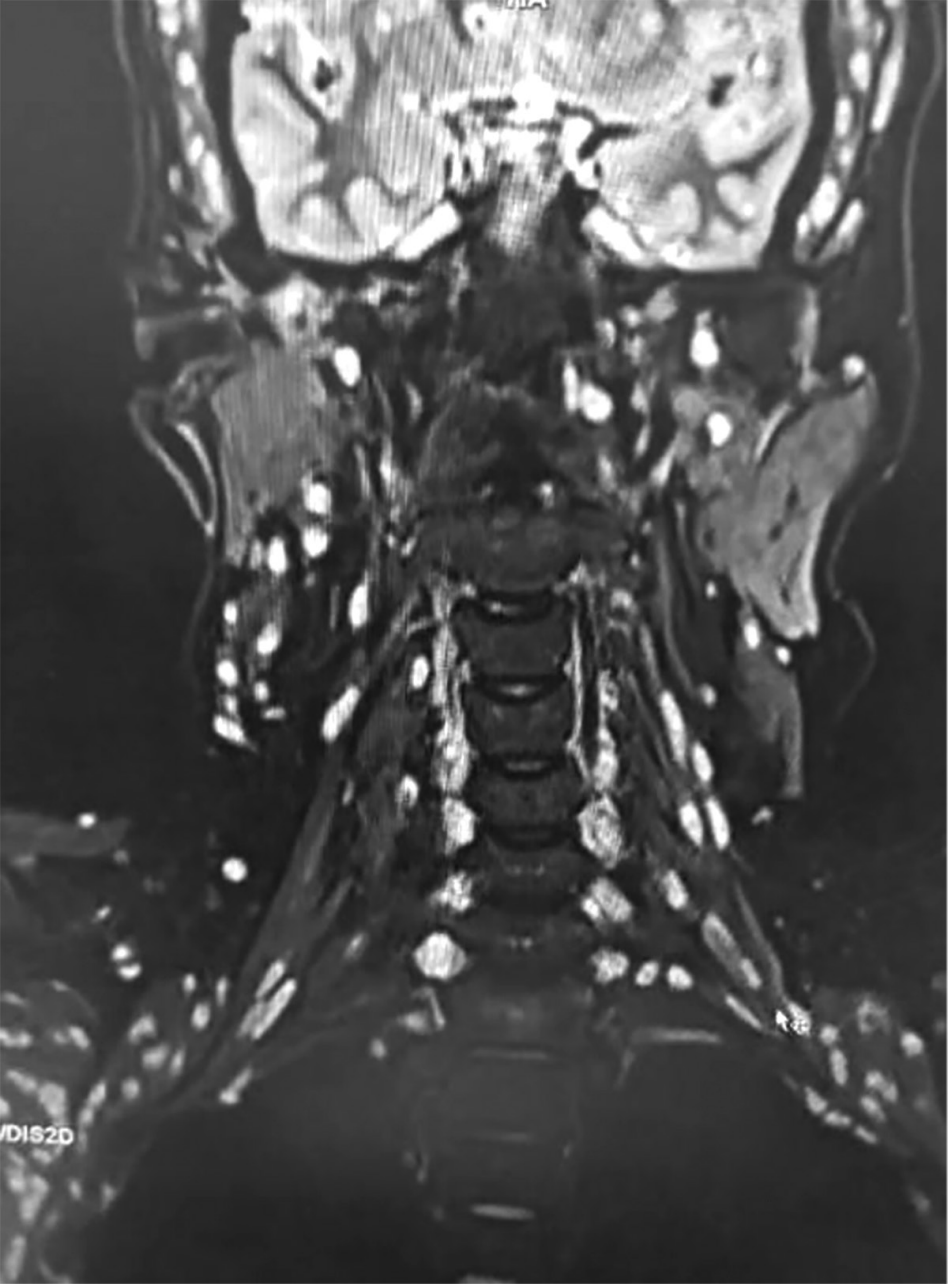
Description: Coronal T2-weighted MRI of the cervical spine and neck muscles shows multiple and well-defined cystic lesions distributed symmetrically within the paravertebral and posterior cervical musculature. Several lesions demonstrate an eccentric hyperintense focus representing the scolex, consistent with myocysticercosis. The cervical vertebral bodies and intervertebral discs are preserved, and no compressive myelopathy is seen. Findings are characteristic of disseminated muscular cysticercosis involving the cervical region. Clinical Significance: This imaging highlights the disseminated muscular form of cysticercosis, which may coexist with neurocysticercosis. Recognition of scolex within cysts on MRI is pathognomonic, allowing differentiation from other soft tissue cystic lesions or abscesses. Preservation of vertebral integrity and absence of cord compression emphasize the importance of systemic antiparasitic therapy over surgical intervention in this case. Origin: @GSVM Medical College, PMSSY Superspeciality Hospital, Kanpur, Uttar Pradesh, India. Pincode: 208002.
